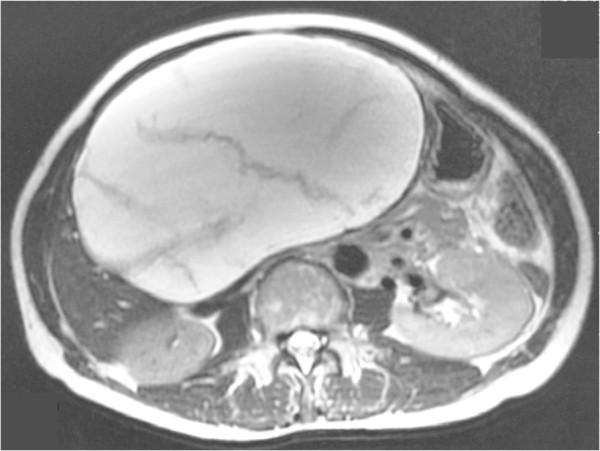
Preoperative findings of T2-weighted magnetic resonance imaging (case 2). A cystic lesion, 17 cm in diameter, occupies almost half of the abdominal cavity on the slice shown. Septum-like structures are seen in the lesion.
